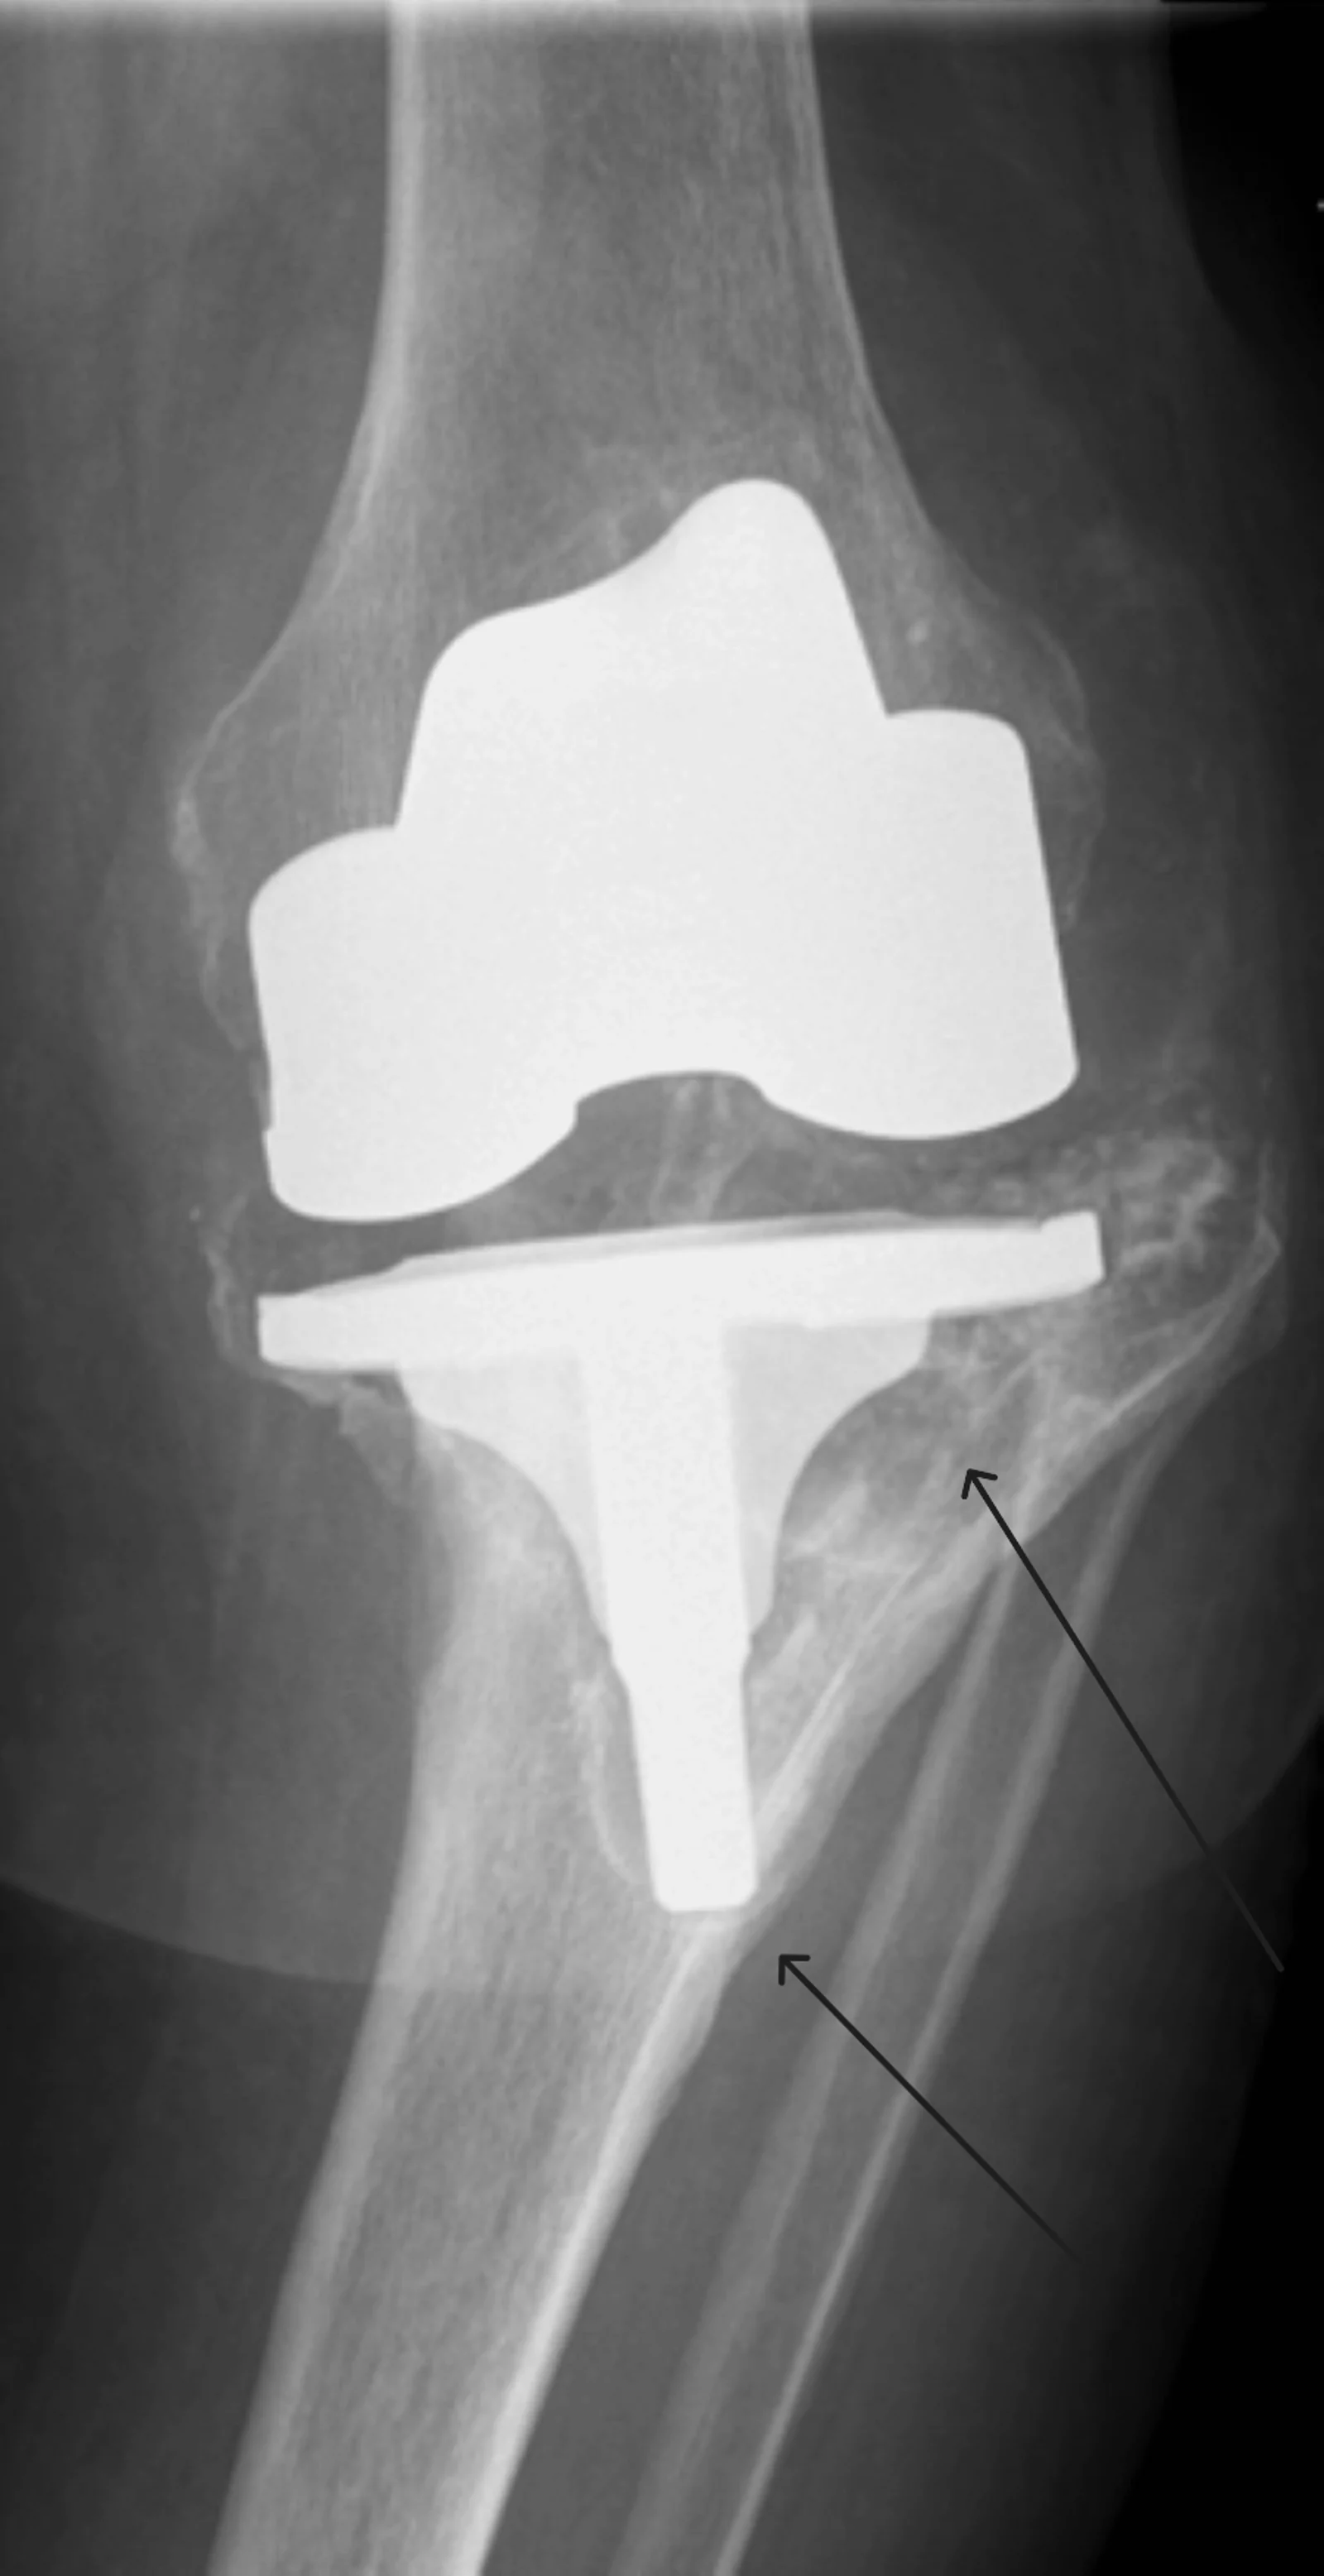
Anteroposterior radiograph of the left knee showing osteolysis of the proximal tibia approximately nine years after primary TKA. TKA, total knee arthroplasty
